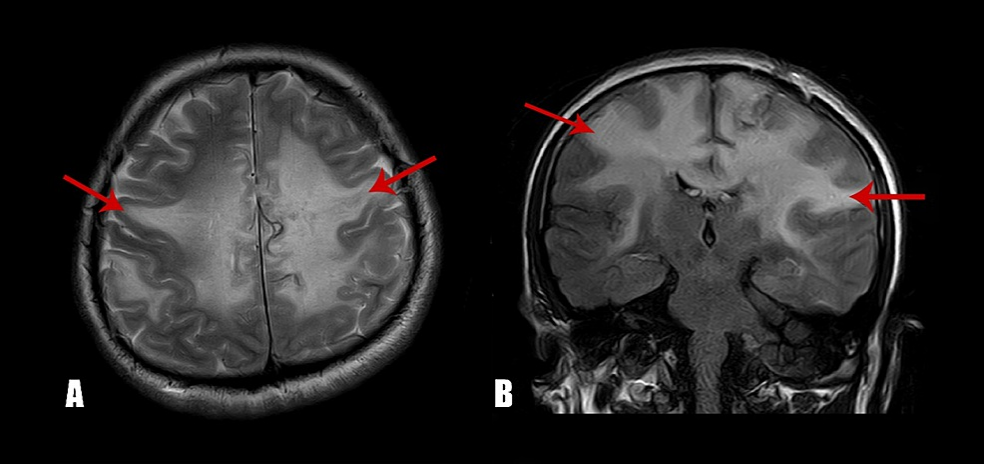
Magnetic resonance imaging of the brain at the time of diagnosis. (A) Axial, T2 section showing abnormal signaling in bilateral white matter consistent with primary disease. (B) Coronal view showing extensive areas of T2/FLAIR hyperintense signal abnormality identified in the supratentorial region involving bilateral cerebral hemispheres. FLAIR: Fluid-attenuated inversion recovery
